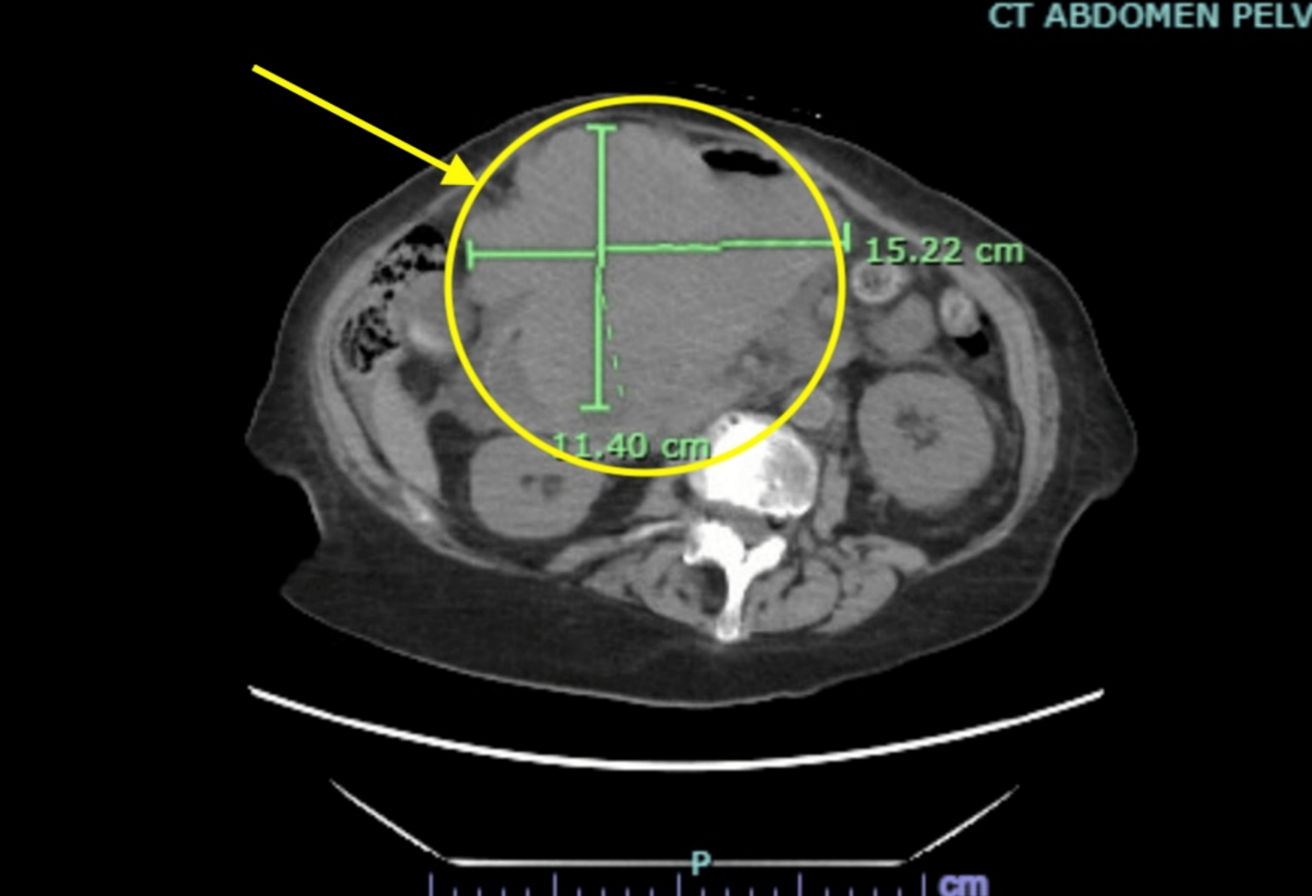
Axial view of the initial CT of the chest, abdomen, and pelvis. The image displays an axial cross-section from the patient’s CT scan of the chest, abdomen, and pelvis, obtained in the emergency department due to elevated liver function tests (see Table 2). The yellow circle and arrow indicate a large abdominal mass measuring 11.40 cm × 15.22 cm.
